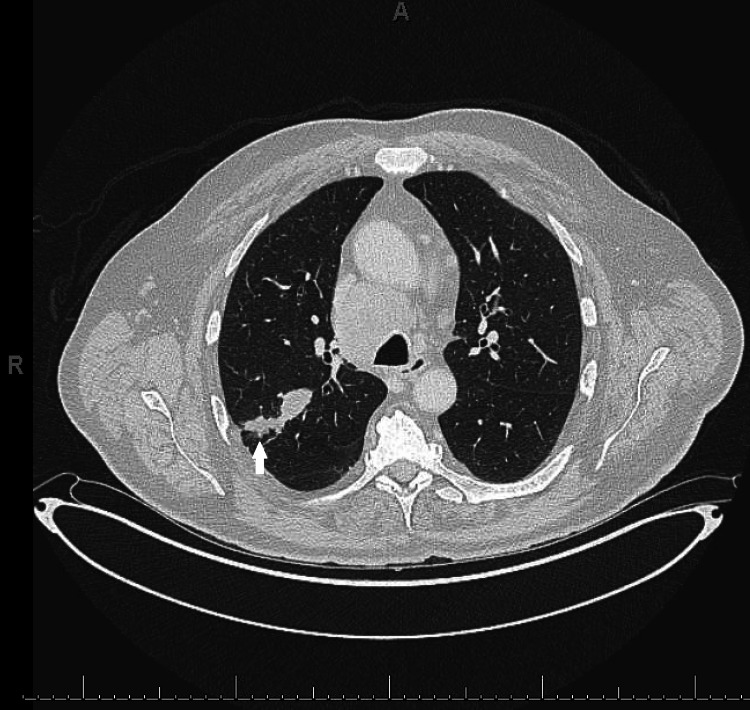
CT chest abdomen pelvis. Showing elongated, irregular, spiculated mass in the right upper lobe, measuring 5x1.6 cm in greatest axial dimension (white arrow).
